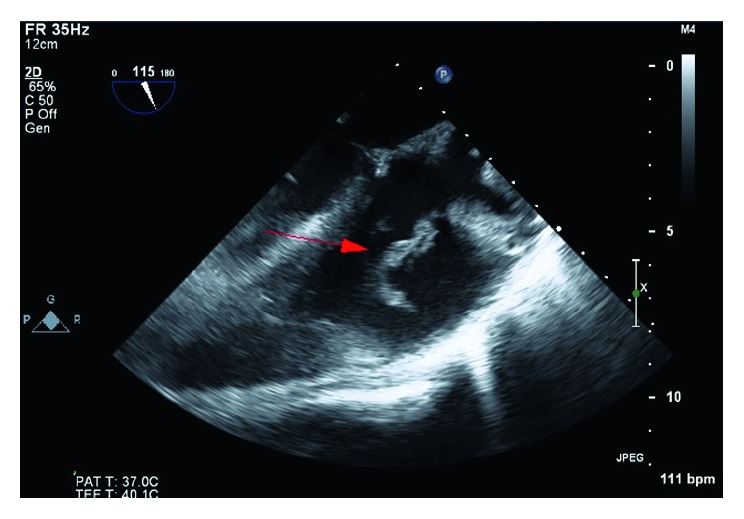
Preprocedural transesophageal echocardiogram (TEE). Midesophageal longitudinal-axis 115-degree view revealing the tricuspid valve vegetation 4.2 cm in its largest diameter (red arrow).
